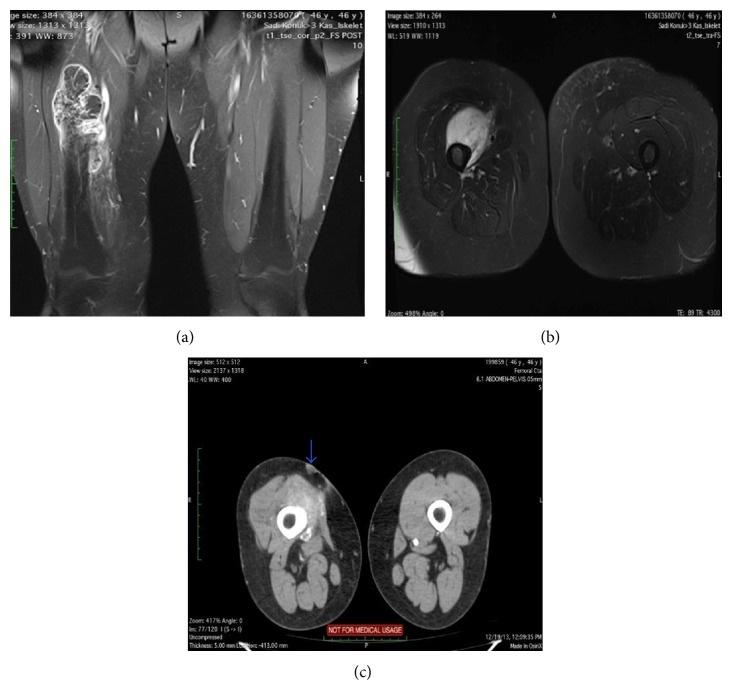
(a) MRI demonstrating tumoral lesion (coronal). (b) MRI demonstrating tumoral lesion (axial). (c) MRI demonstrating cutaneous relapse.
